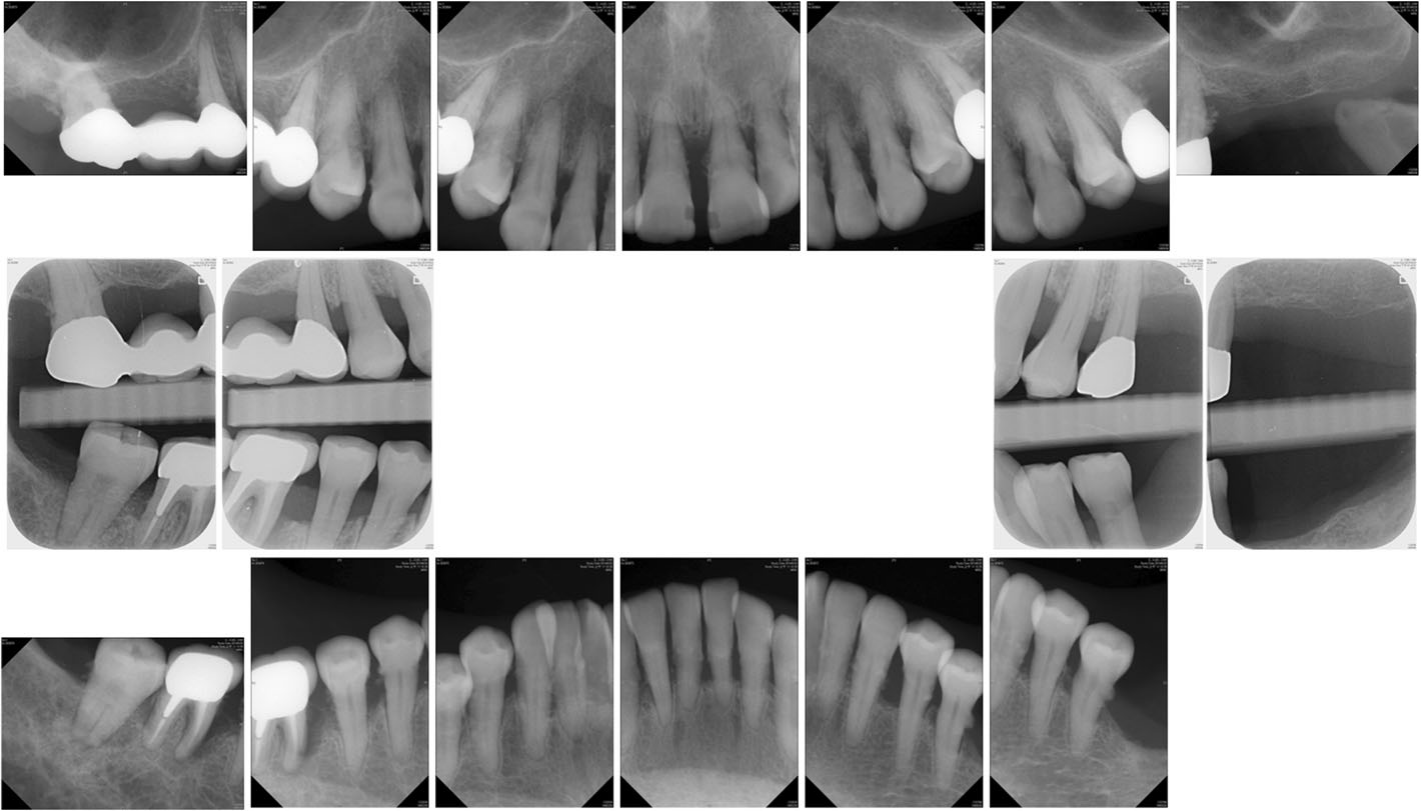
Initial radiographs. Note the bone loss over mesial root of the lower right first molar and multiple subgingival deposits
